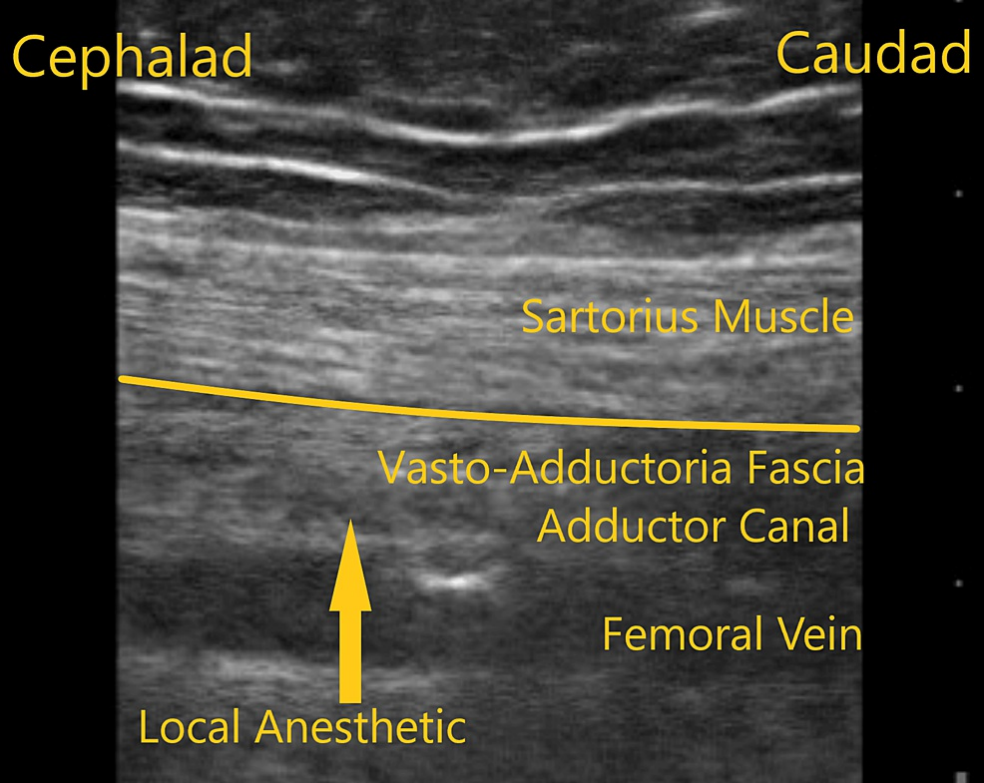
Ultrasound scan image along the sagittal plane of the adductor canal demonstrating only adductor canal spread of local anesthetic.
